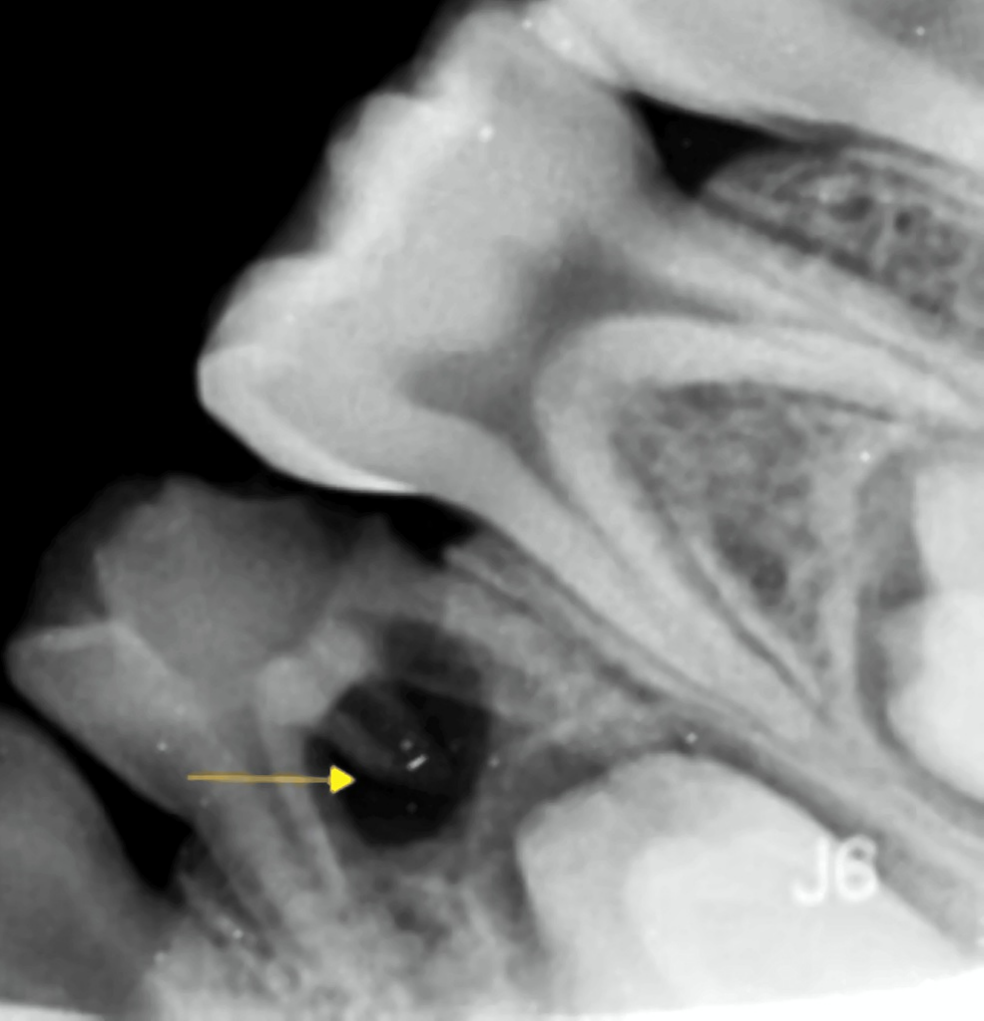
Depiction of the preoperative radiograph of RE of the right deciduous mandibular first molar RE: Radix entomolaris
